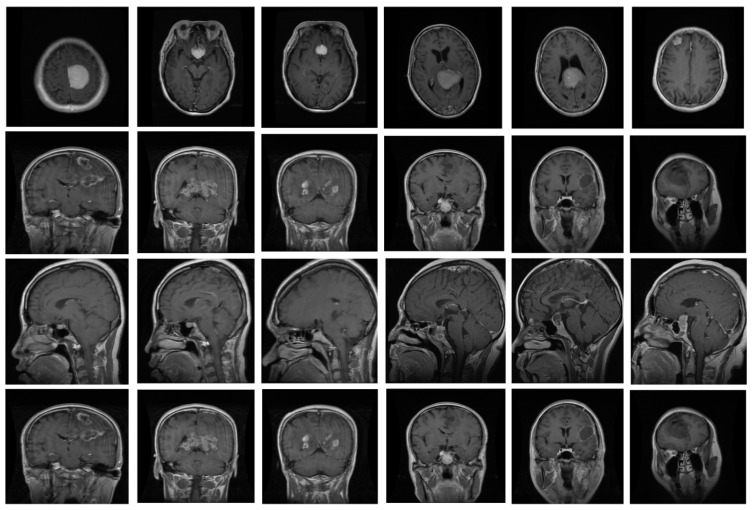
Some samples of brain MRI.
